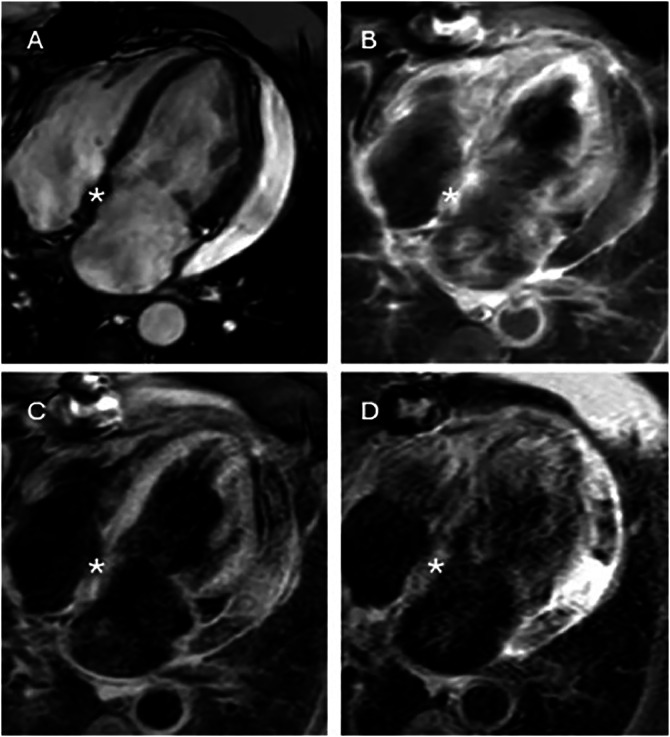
Cardiac magnetic resonance (CMR) images postsurgery: (A) SSFP 4-chamber view shows a remaining thickening of the atrial septum at the previous location of the mass (*). (B) Short tau inversion recovery (T2 STIR) 4-chamber view demonstrating slightly high signal in the same area. (C) T1-weighted sequence without contrast showing heterogeneous diffuse high signal. (D) T1-weighted sequence with contrast slightly diffuse inhomogeneous gadolinium enhancement. Note that there is also pericardial effusion.
